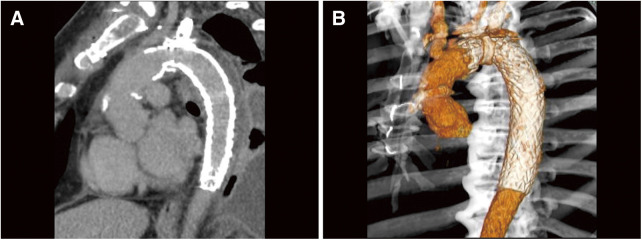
Fig. 3 Enhanced CT imaging (A, B) after TEVAR, revealing the absence of an endoleak and the good expansion of the stent graft. CT: computed tomography; TEVAR: thoracic endovascular aortic repair
